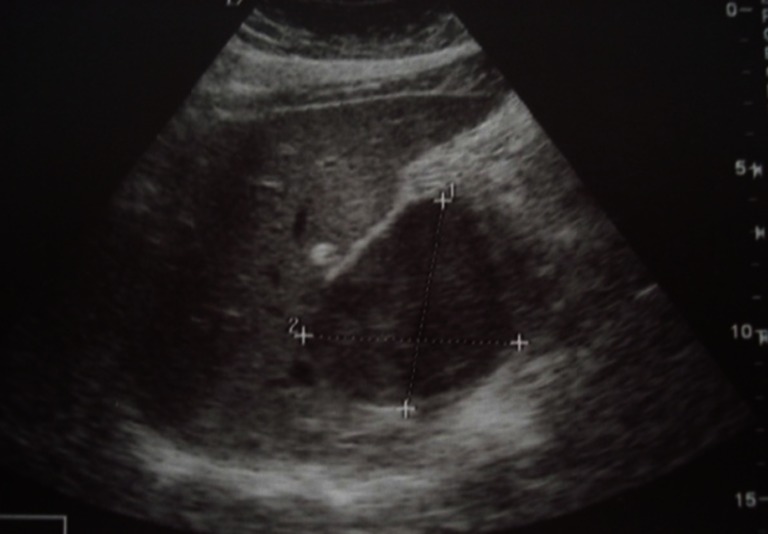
Abdominal ultrasound image showing a hypo-echoic mass developed in segment I of the liver, with regular outlines
